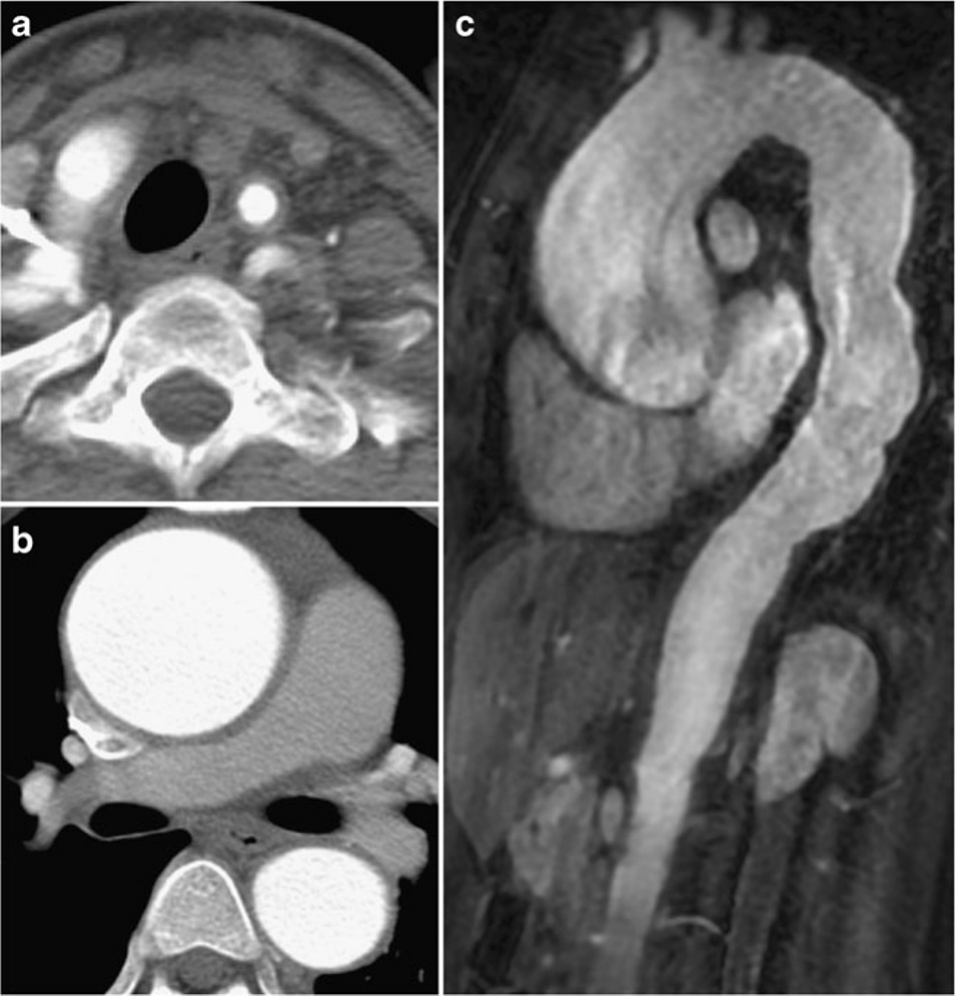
a–c 20-year-old previously healthy woman with chest pain. CTA of the lower neck demonstrates circumferential wall thickening of the left carotid artery (a). Ascending aorta is dilated with circumferential wall thickening (b). Gadolinium-enhanced sagittal (c) view demonstrated dilatation of both ascending and descending thoracic aorta as well as aortic wall thickening including in the abdominal aorta
